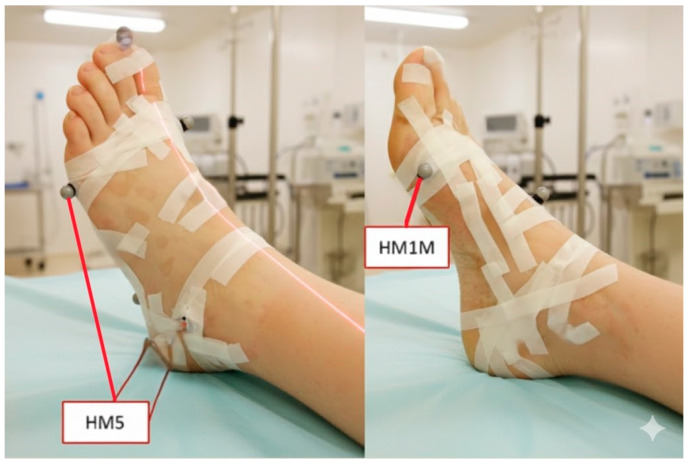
The placement of markers (HM1M and HM5) for radiographic measurement [25].
